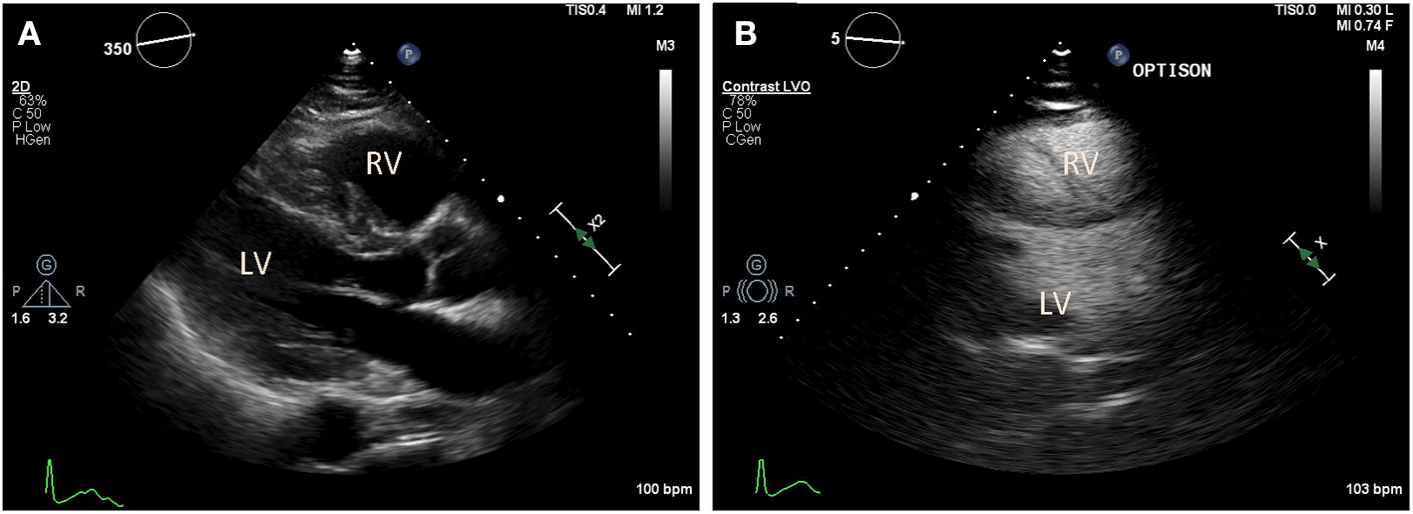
Transthoracic echocardiography (TTE) in a COVID-19 positive patient who presented with acute bilateral pulmonary emboli and acute right sided heart failure requiring emergent veno-venous extracorporeal membrane oxygenation (VV-ECMO) cannulation. (A) Parasternal long axis view demonstrate a McConnell's sign: Right ventricle (RV) enlargement and hypokinesis with preserved apical contractility. (B) Optison enhanced images demonstrate RV enlargement, and paradoxical septal motion consistent with RV dysfunction.
